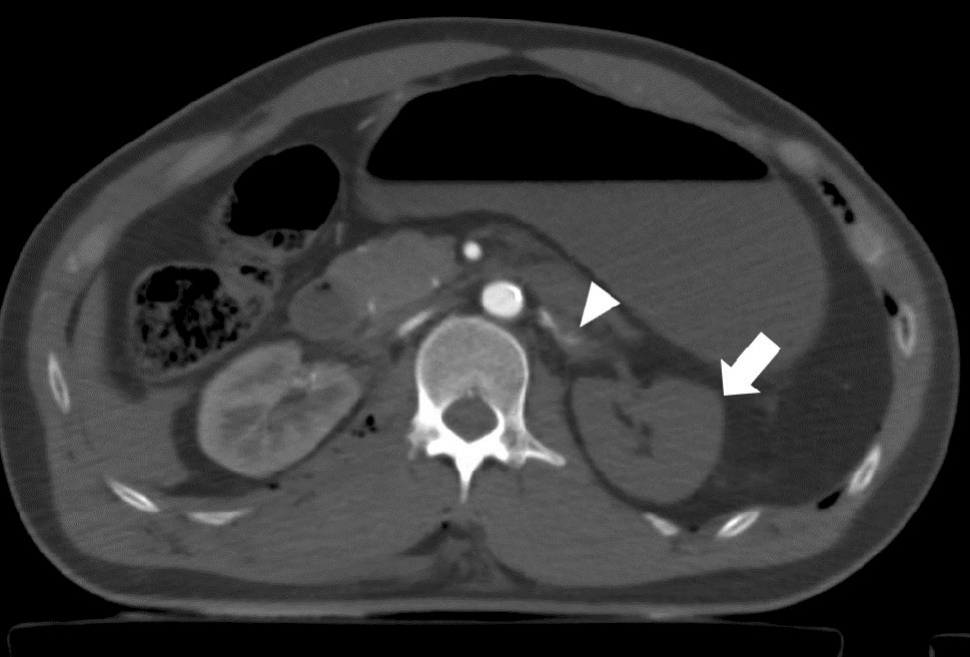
Abdominal contrast-enhancement computed tomography with partial resuscitative endovascular balloon occlusion of the aorta in Zone I in a patient with multiple traumas showing no enhancement in the left kidney (arrow). The left renal artery was irregular and occluded (vascular injury) (arrowhead)
